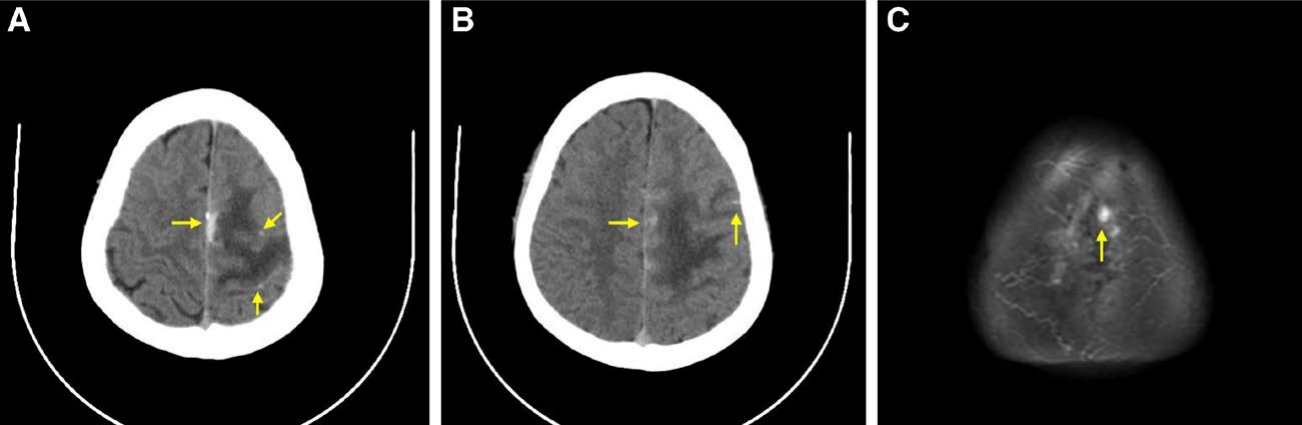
Images showed SAH and high signal in frontal bone plate. (A and B) CT scan showed left frontal lobe and interhemispheric cistern SAH with edema. (C) MRI showed high signal in left frontal bone plate on FLAIR. CT = computed tomography, FLAIR = fluid attenuated inversion recovery, MRI = magnetic resonance imaging, SAH = subarachnoid hemorrhage.
